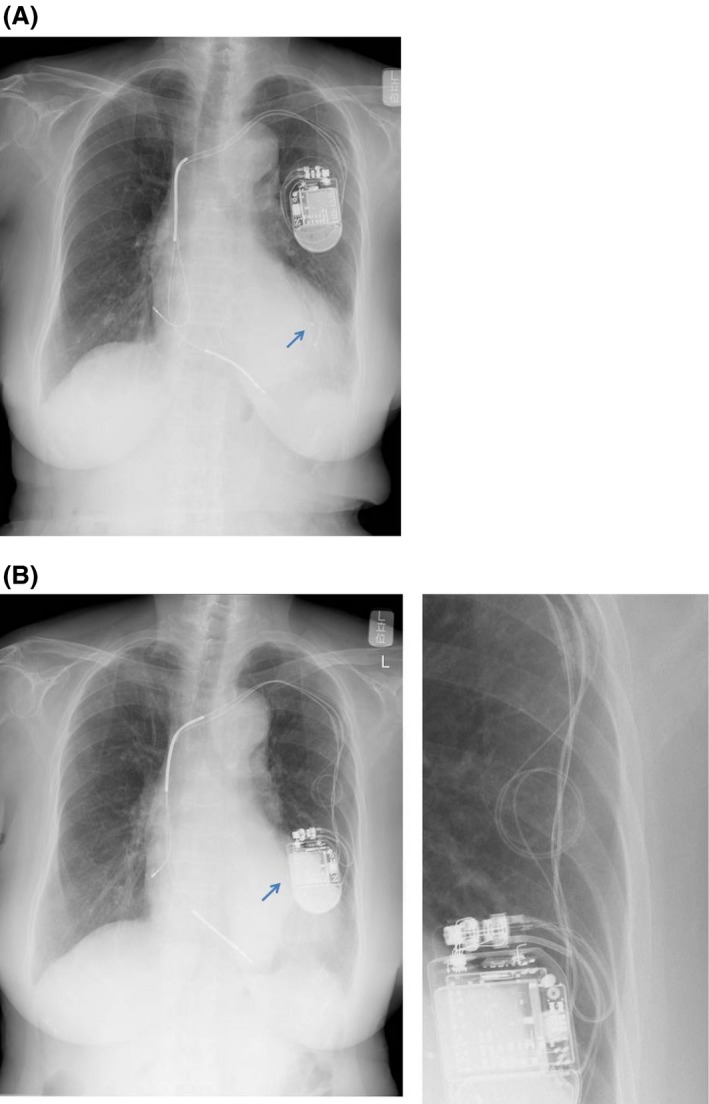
The CIED was located at the correct site just after implantation (A). However, chest radiography 1 year after implantation demonstrated dislodgement of the leads and generator (B). All leads had been pulled. Arrows point the LV lead's tips, which had been pulled proximal direction. Enlarged picture shows twisted leads.
